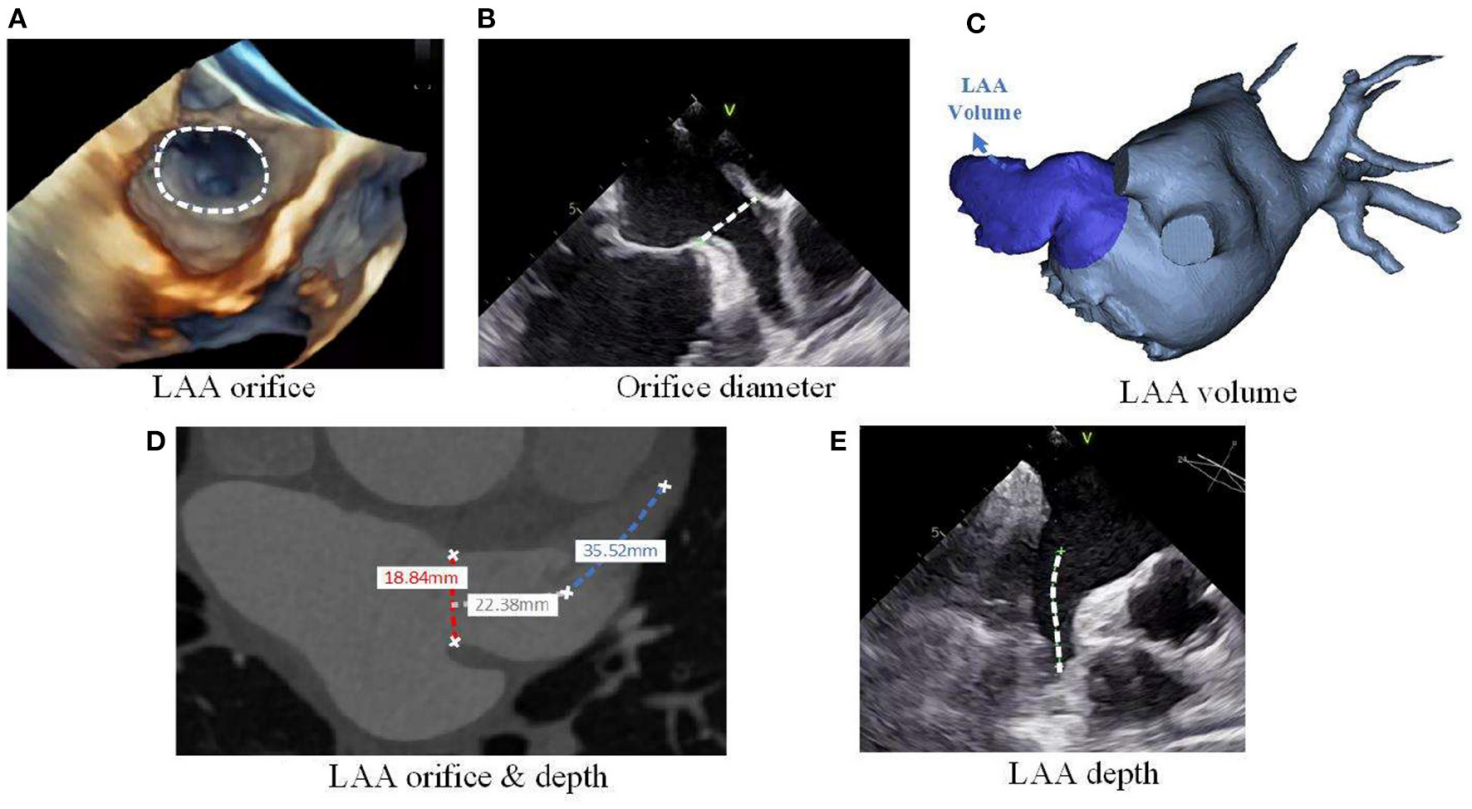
LAA morphological parameters. (A) LAA orifice measurement from 3D echo, (B) LAA orifice diameter measurement from planer echo, (C) LAA volume measurement from 3D reconstruction of CT images, (D) LAA orifice & depth measurement from planer CT images, and (E) LAA depth measurement from planar echocardiogram.
